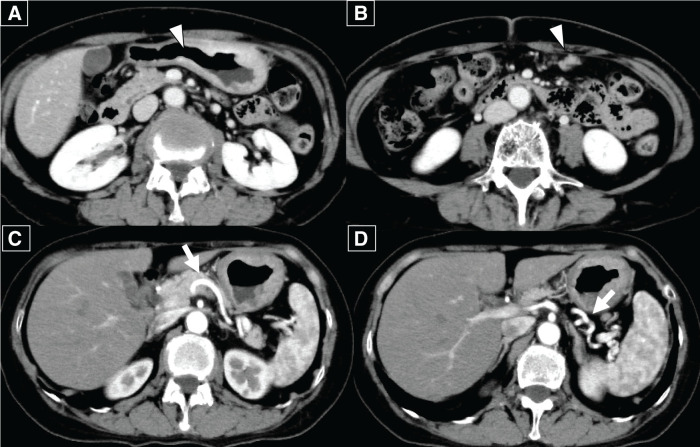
(A, B) Preoperative contrast-enhanced abdominal CT scan showed wall thickening with enhancement at the gastric angle and an enlarged lymph node along the greater curvature (white arrowhead). (C, D) A highly tortuous splenic artery was observed (white arrow).
